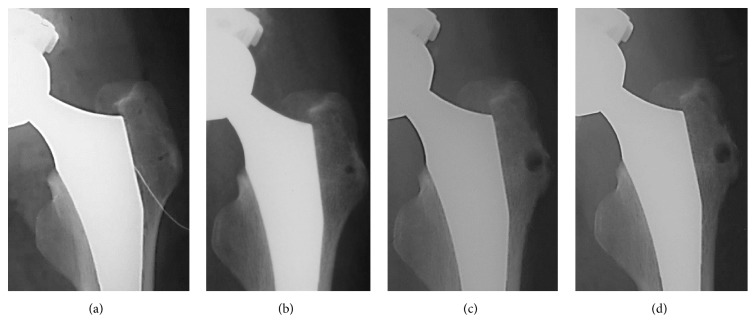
Radiographs of the left hip joint after THA. (a) The initial radiograph after THA. (b) At 4 weeks after THA. (c) At 8 weeks. (d) At 10 weeks.
